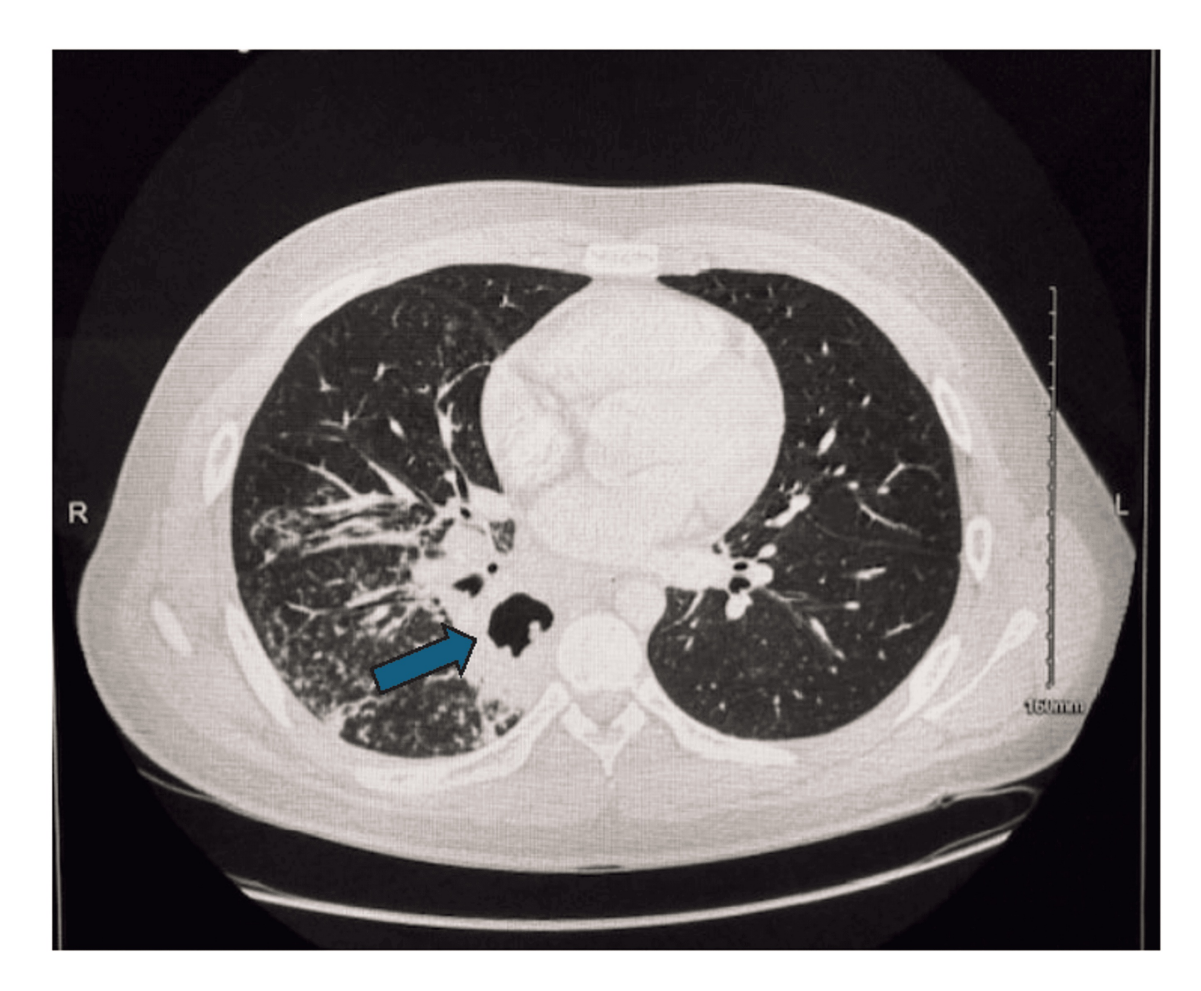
CT thorax two weeks later CT thorax with contrast two weeks after initial imaging demonstrating persistent but improved multifocal pneumonia with cavitation.
